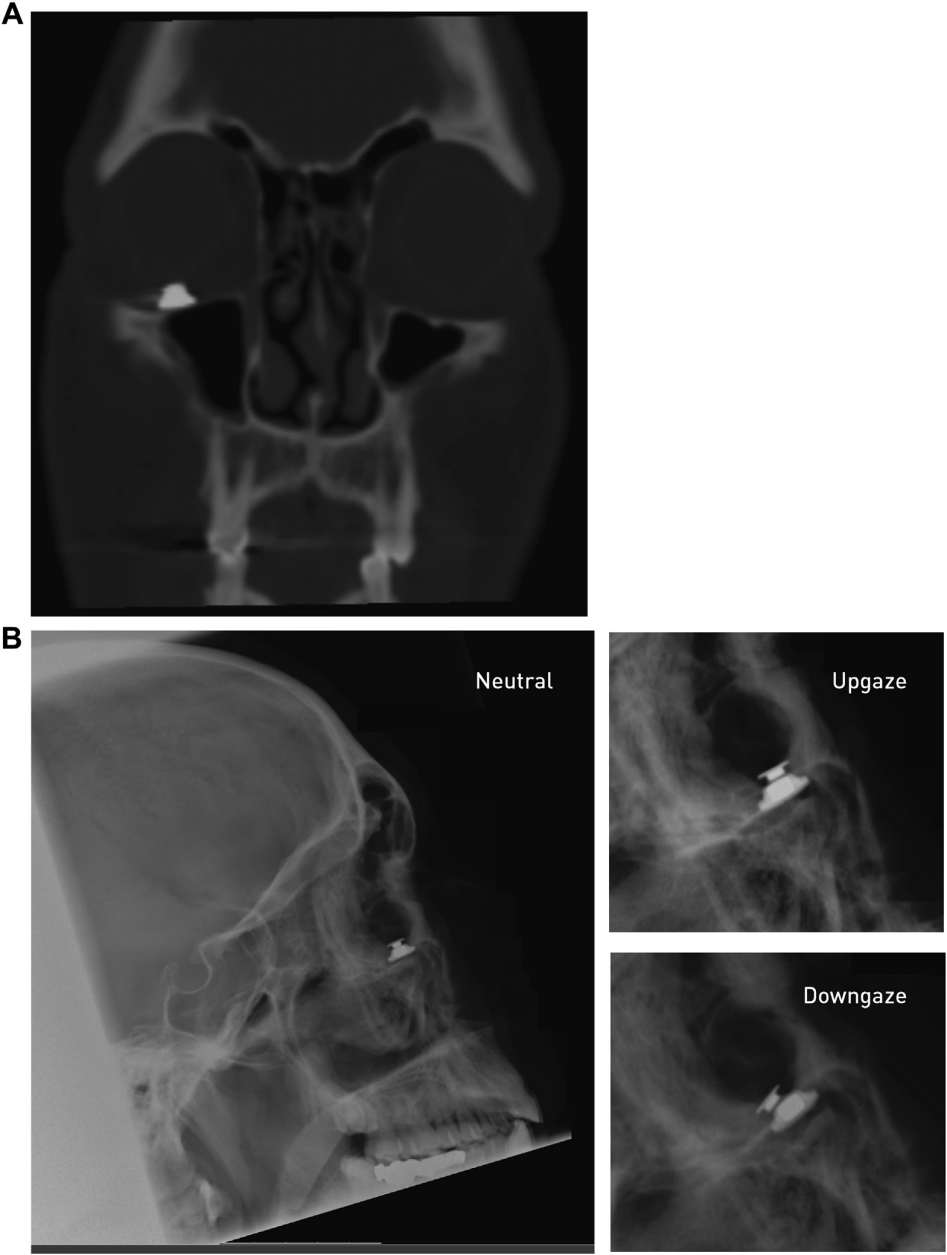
A, Orbital computer tomographic image showing the position of the right implant in the coronal plane. Note that the space between the 2 components is not visible because of artefact. B, Lateral plain radiograph showing the position of the right implant in the sagittal plane in 3 positions of gaze: neutral, upgaze, and downgaze. Note the movement of the eye component relative to the orbital component.
